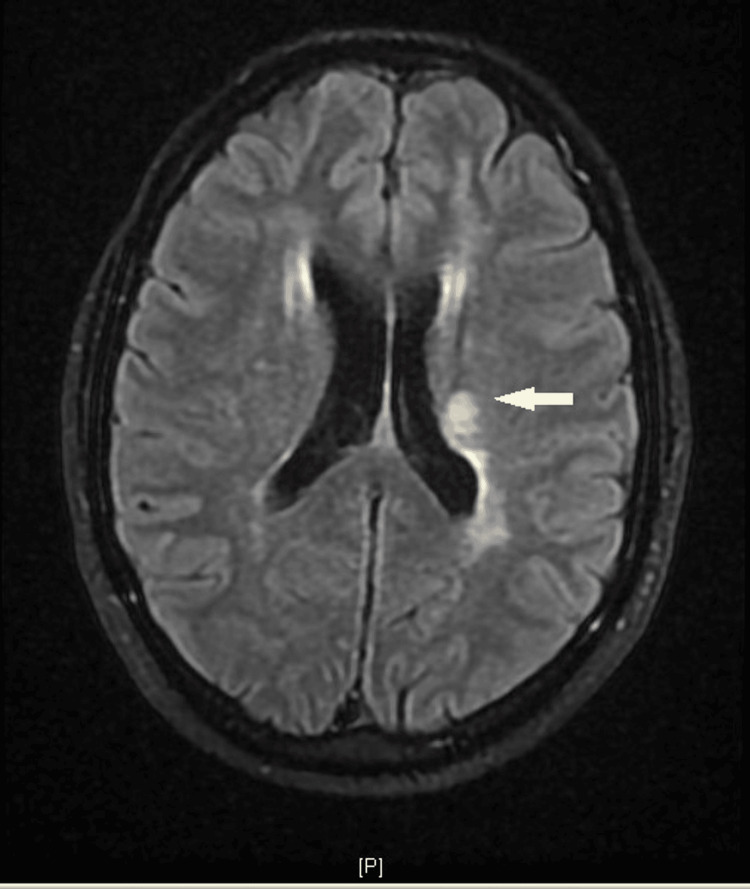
Brain MRI showing ischemic lesion in the left posterior lenticulocapsular region irrigated by the left thalamocapsular arteries
